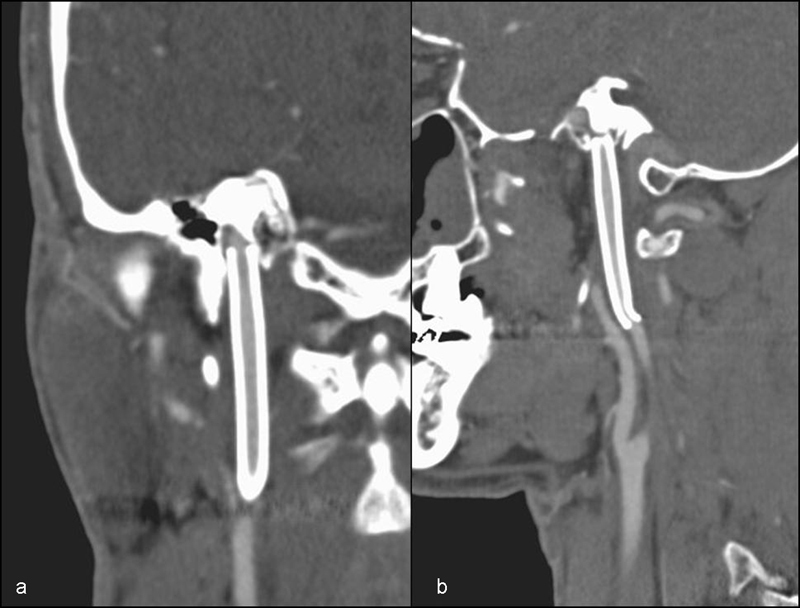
Coronal (a) and sagittal (b) computed tomography angiography scans during follow-up after 6 months shows absence of pseudoaneurysm.
